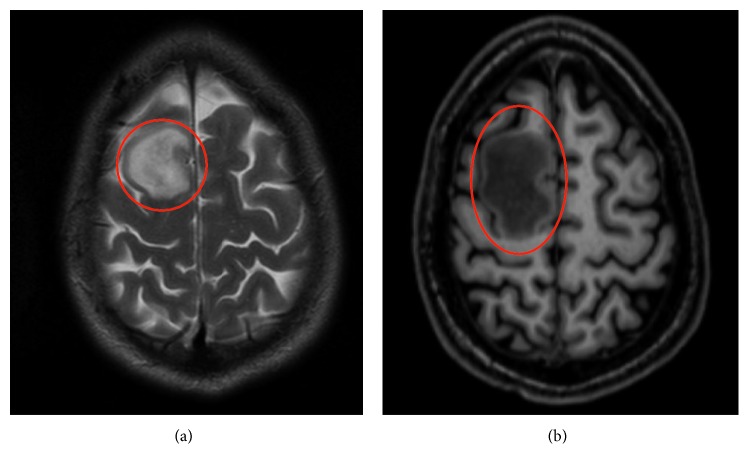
Cranial MRI in T2 and T1, respectively—frontal lesion corresponding to PCNSL.
